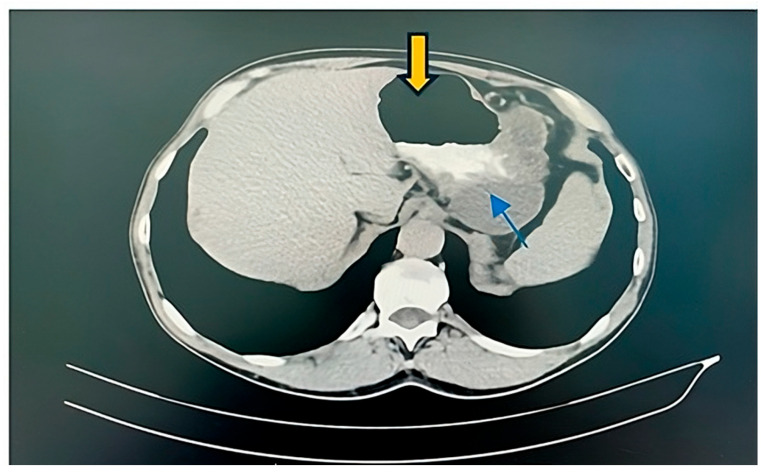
CT image: A clearly outlined peri-gastric hypoattenuating polylobate lesion (blue arrows) is seen adjacent to the great curvature of the stomach (yellow arrow), with a thin wall, homogeneous content and the contrast outlet only at the parietal level; its dimensions are approx. 102/80/60 mm. (Personal collection).
